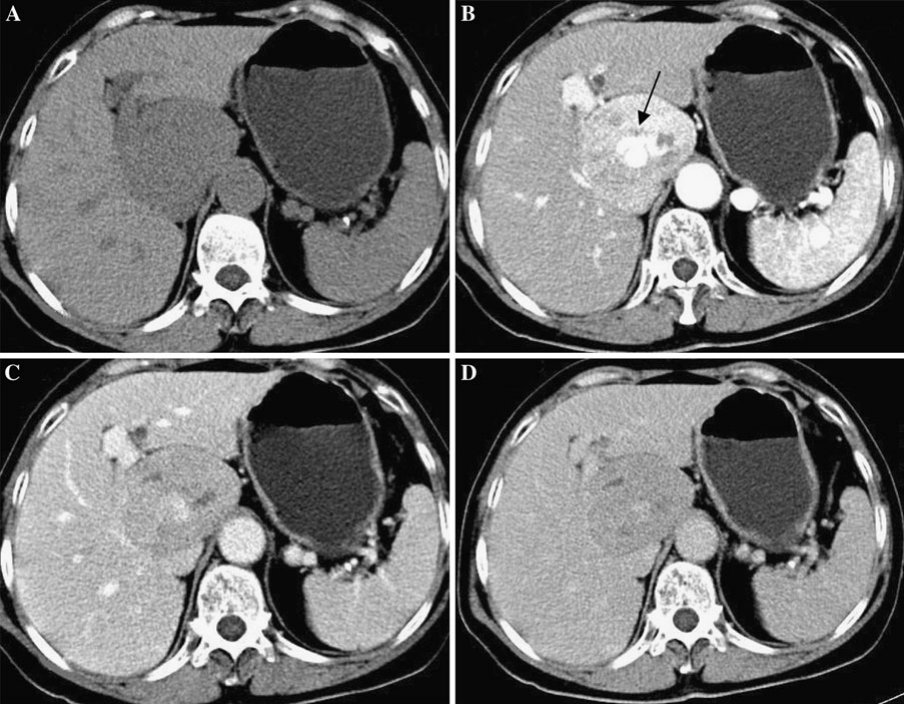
64-Year-old woman with epithelioid AML in caudate lobe of liver (patient 1). A Non-enhanced CT scan shows hypoattenuating lesion in segment. B Contrast-enhanced CT scan shows inhomogeneous enhanced lesion with thickly distorted vessels (*arrow*) in the arterial phase. C, D The lesion is hypoattenuating in the portal venous/delayed phase.
